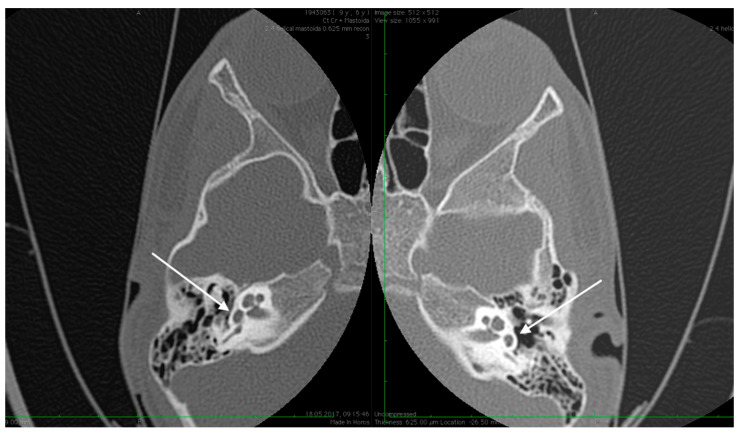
High resolution axial CT scan of left temporal bone showing a missing oval window and stapes suprastructure (arrow) on both sides.
